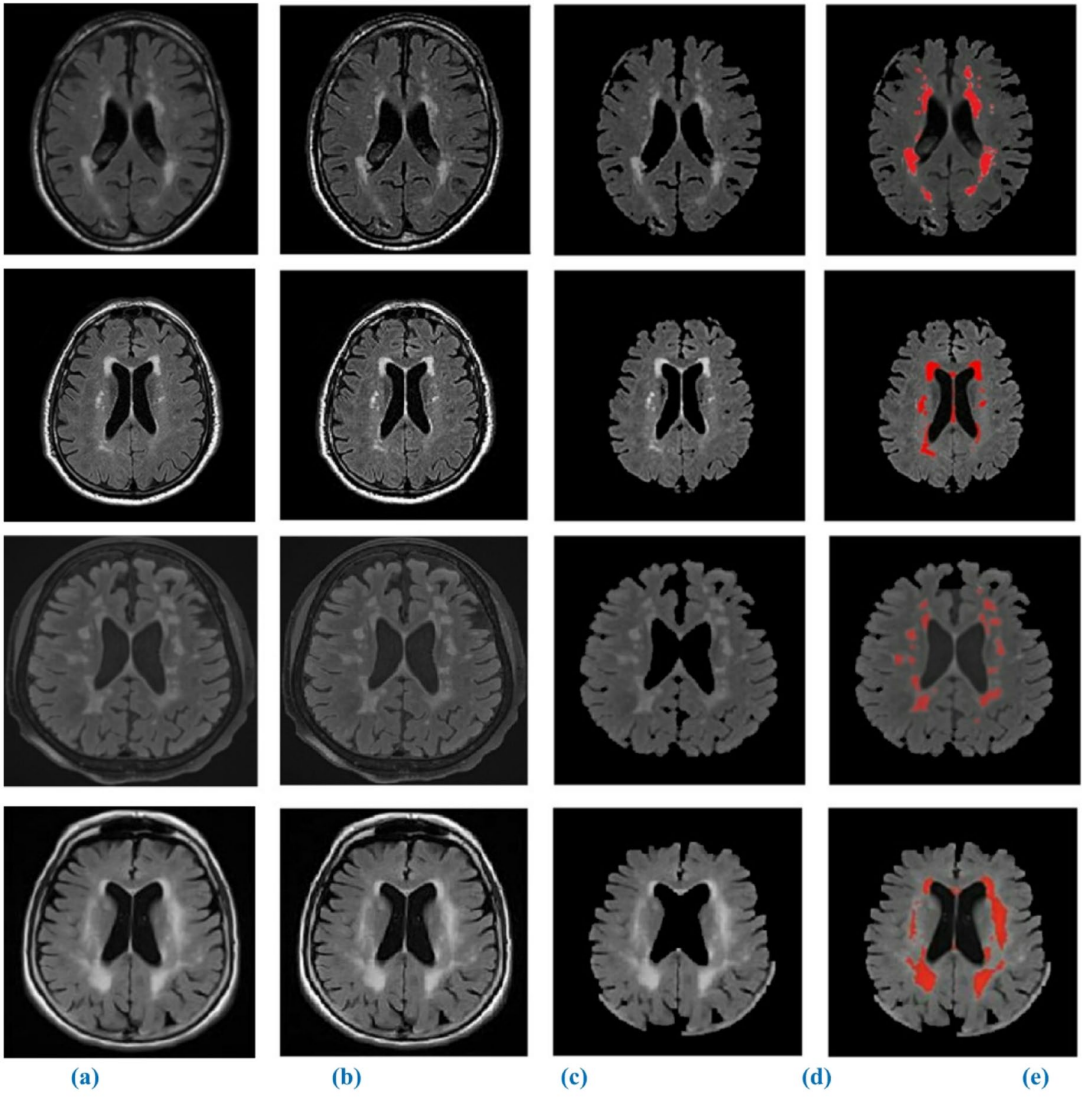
Segmentation of WMH on high-resolution T2-w MRI images. (a) original image, (b) enhanced image (c) skull stripping, (d) binary mask image, (e) Segmented output.
